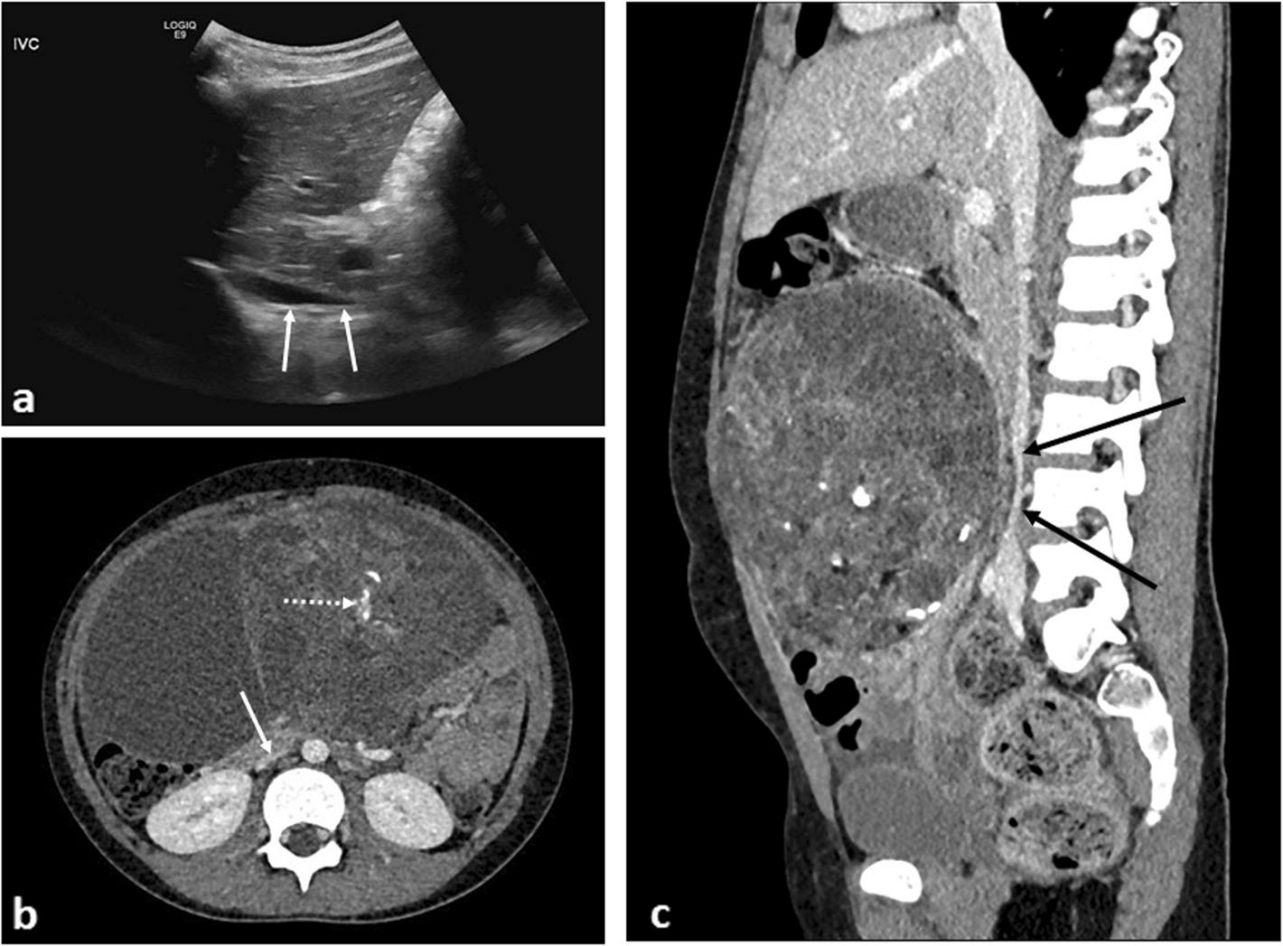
An 8-year-old girl with increasing abdominal distension secondary to a malignant germ cell tumour (MGCT). a A sagittal abdominal ultrasound image demonstrates tapering of the upper abdominal inferior vena cava (IVC) (white arrows). b Axial contrast-enhanced CT image at the level of the kidneys demonstrates flattening of the IVC (arrow). There is also a large heterogenous mass in the anterior abdomen containing internal foci of calcification (dashed arrow). c Sagittal CT image of the IVC reveals demonstrates compression of the vessel (black arrows), again placing the patient at risk of poor central venous return
